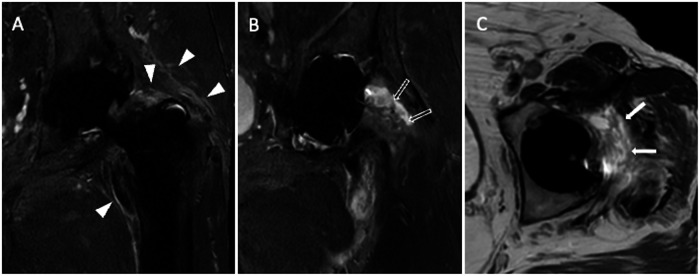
Another case of infection of the left THA of a 69-year-old male. Coronal STIR (A, B) images show pericapsular edema (white arrowheads, A), effusion, and synovitis (void arrows, B). Axial T2-weighted (C) image shows lamellated synovitis (arrows) close to the prosthetic neck
